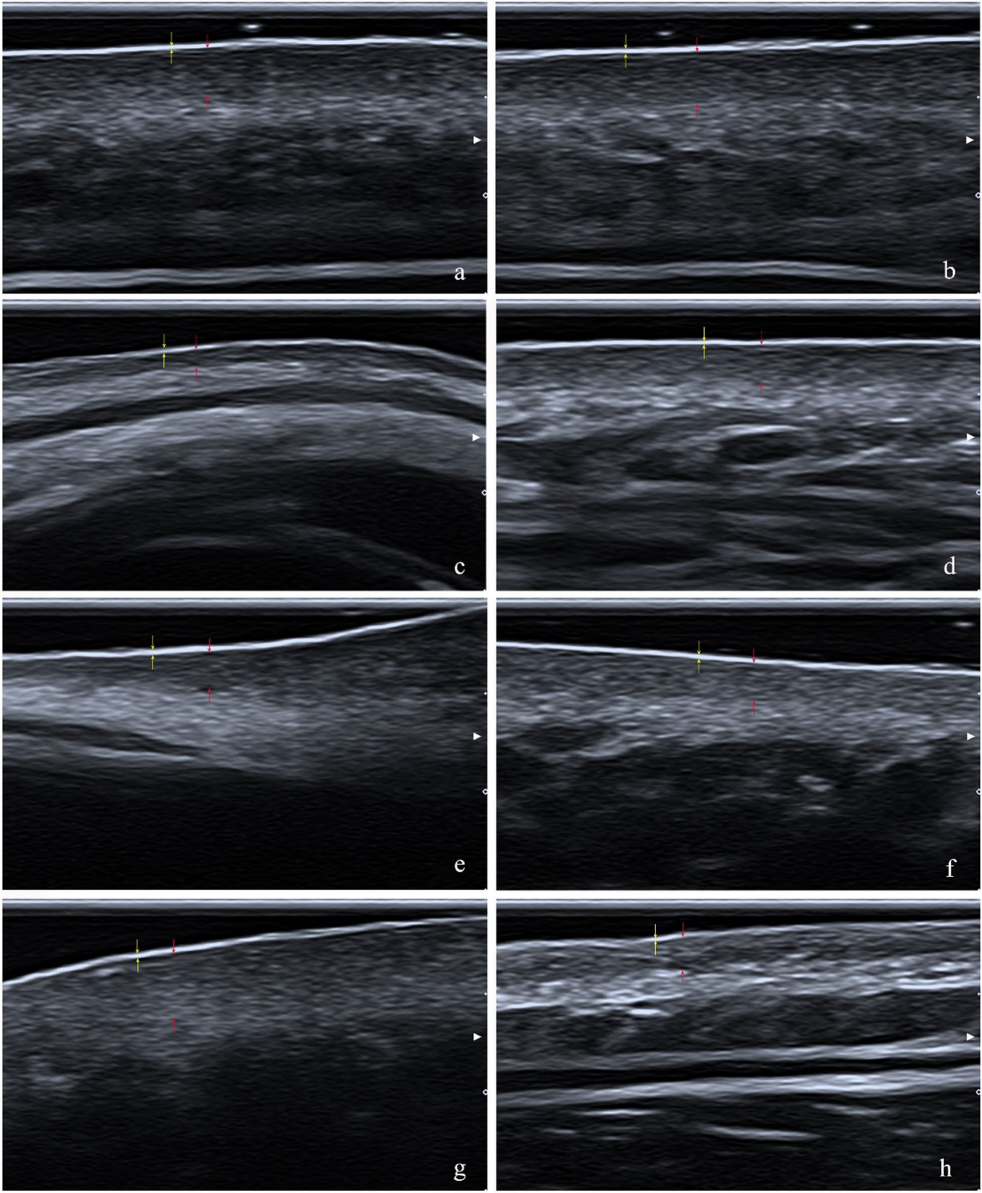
Ultrasound images of dermis and epidermis at different facial sites. Yellow arrow is epidermal thickness; Red arrow is dermal thickness. a forehead; b glabella; c temple; d eyelid; e nasal dorsum; f zygoma; g submandibular; h neck
